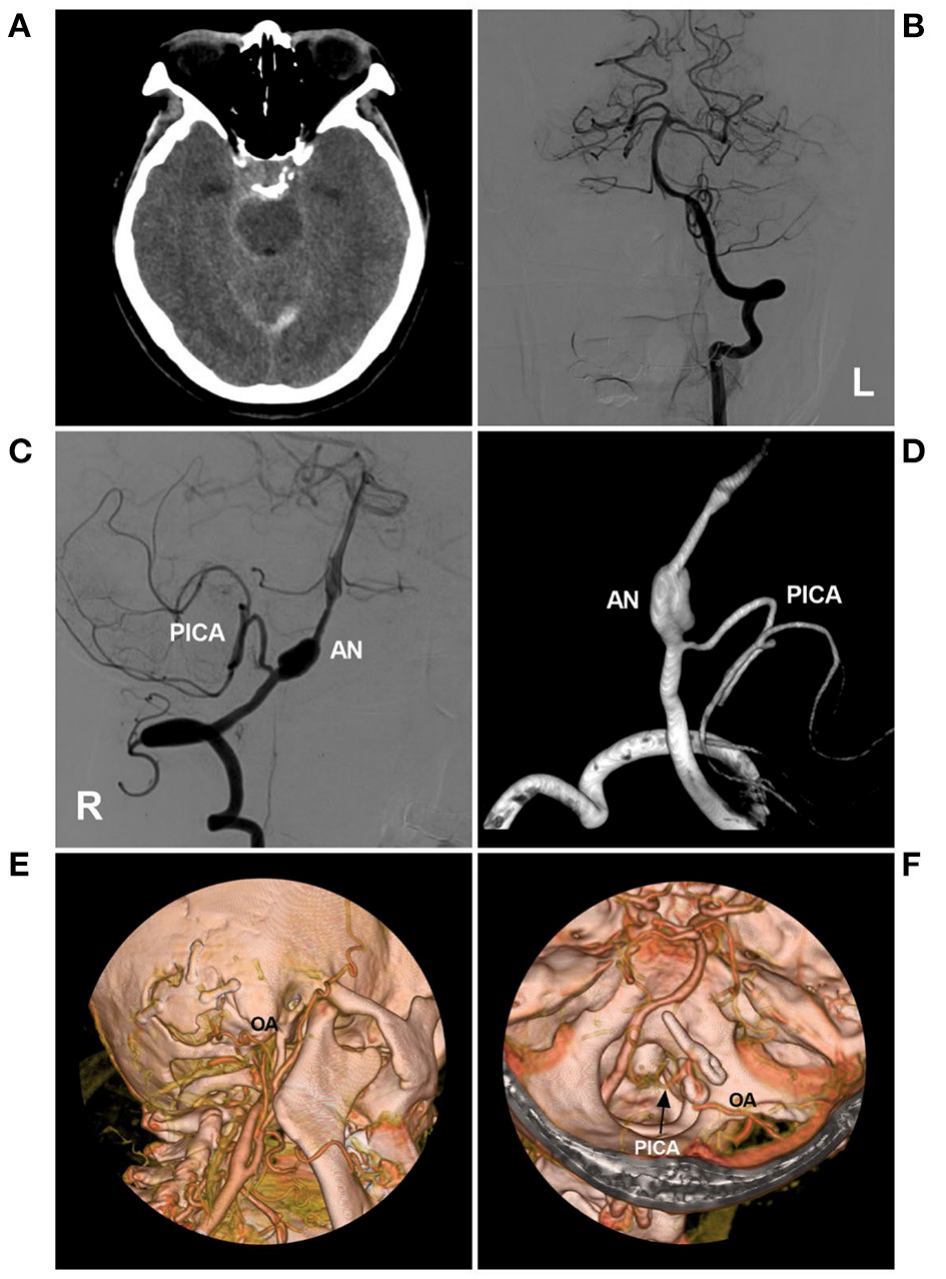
PAO with the assistance of low-flow bypass in a VA fusiform AN. (A) CT showed subarachnoid hemorrhage around the brainstem. (B) DSA of the left VA showed that the VA was normal. (C,D) DSA (C) and three-dimensional DSA (D) of the right VA showed the fusiform AN above the origin of the PICA. (E,F) CTA showed PAO under the assistance of low-flow bypass. (E) the OA entered the cranium through the bone window. (F) the OA was anastomosed with the PICA trunk (arrow), and the AN was trapped with the clips. AN, aneurysm; CT, computed tomography; CTA, computed tomography angiography; DSA, digital subtraction angiography; OA, occipital artery; PICA, posterior inferior cerebellar artery; L, left; PAO, parent artery occlusion; R, right; VA, vertebral artery.
